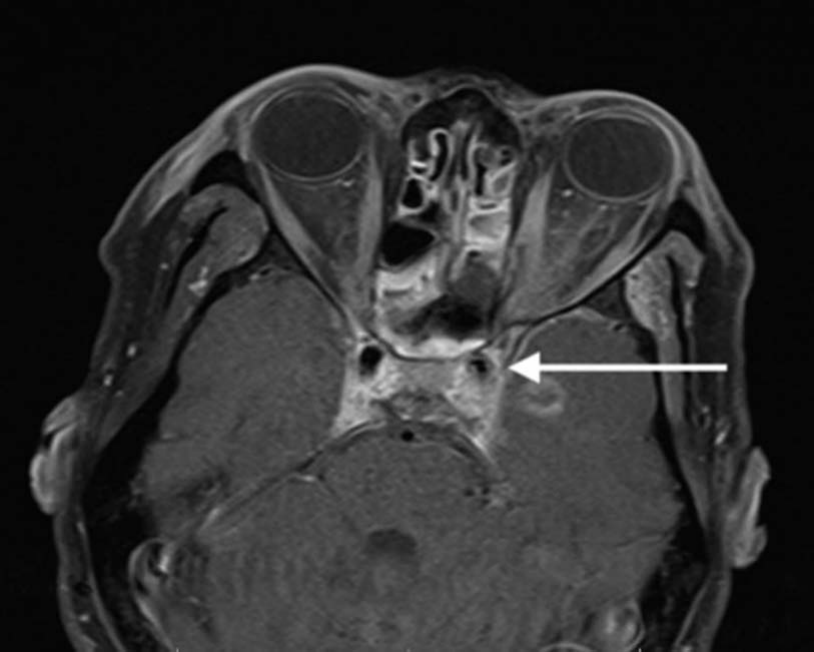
A 48-year-old male presenting with the mild left sided facial numbness and sinusitis. Axial postcontrast T1-weighted image shows heterogeneously enhancing increased signal intensity in the left intraconal space with extension to left cavernous sinus causing luminal narrowing of cavernous segment of left internal carotid artery (white arrow). Bilateral dural enhancement of cavernous sinus and enhancing parenchymal lesion in left temporal lobe is seen.
